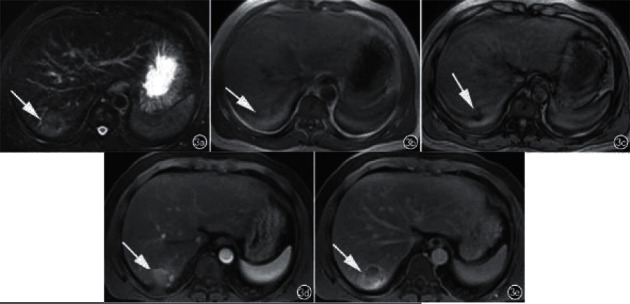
Axial MRI images of a patient with HCC in the right lobe of the liver. (a) A T2 high signal nodule is seen in the right posterior lobe of the liver. (b) The lesion is mostly isosignal on in-phase T1 images, with slightly higher signal (arrows) locally. (c) The lesion is significantly low signal (arrows) on in-phase T1 images, suggesting that the lesion contains lipid. (d) The lesion is significantly enhanced in the arterial phase. (e) Delayed phase contouring.
